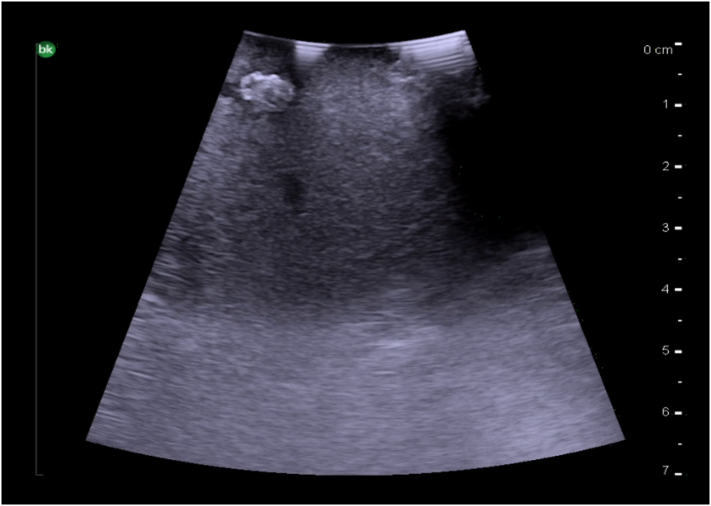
Intraoperative ultrasound appearance of a hemangioma (hyperechoic).
